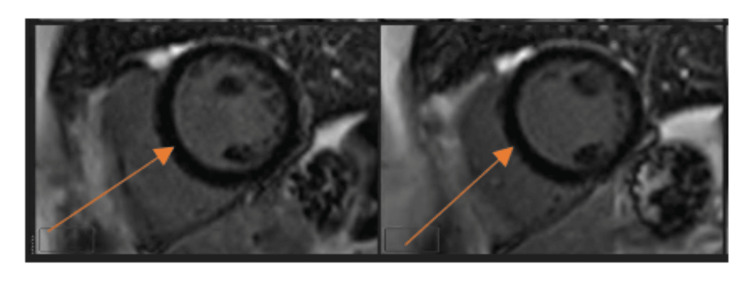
Cardiac magnetic resonance imaging demonstrating normal myocardial tissue characterization. Late gadolinium enhancement images showing no myocardial enhancement (orange arrows), consistent with absence of myocardial fibrosis or scar.
